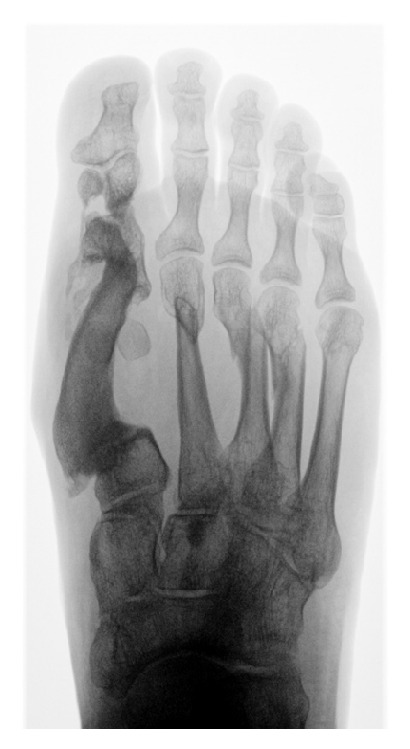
Case 4—final radiograph of consolidated bone graft.
